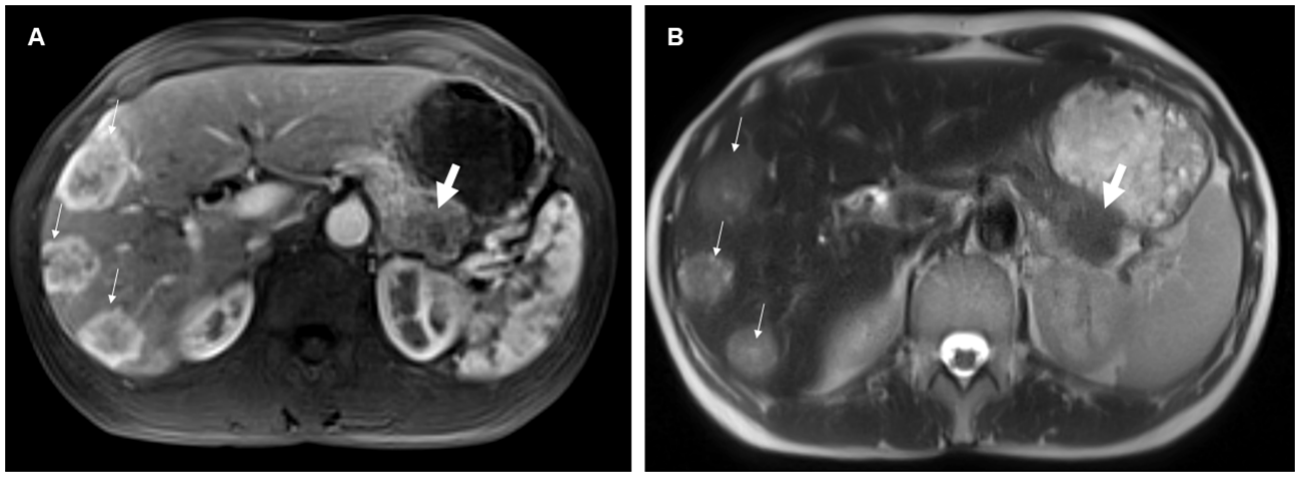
54-year-old female with pancreatic neuroendocrine tumor and synchronous neuroendocrine liver metastases. On contrast-enhanced T1-weighted images in the arterial phase (A), the liver metastases (small arrows) predominantly exhibited hyperenhancement, whereas the pancreatic primary tumor (thick arrow) was hypoenhancing compared to the liver metastases. Additionally, the neuroendocrine liver metastases were more hyperintense on the T2-weighted image (B) compared to the primary tumor.
